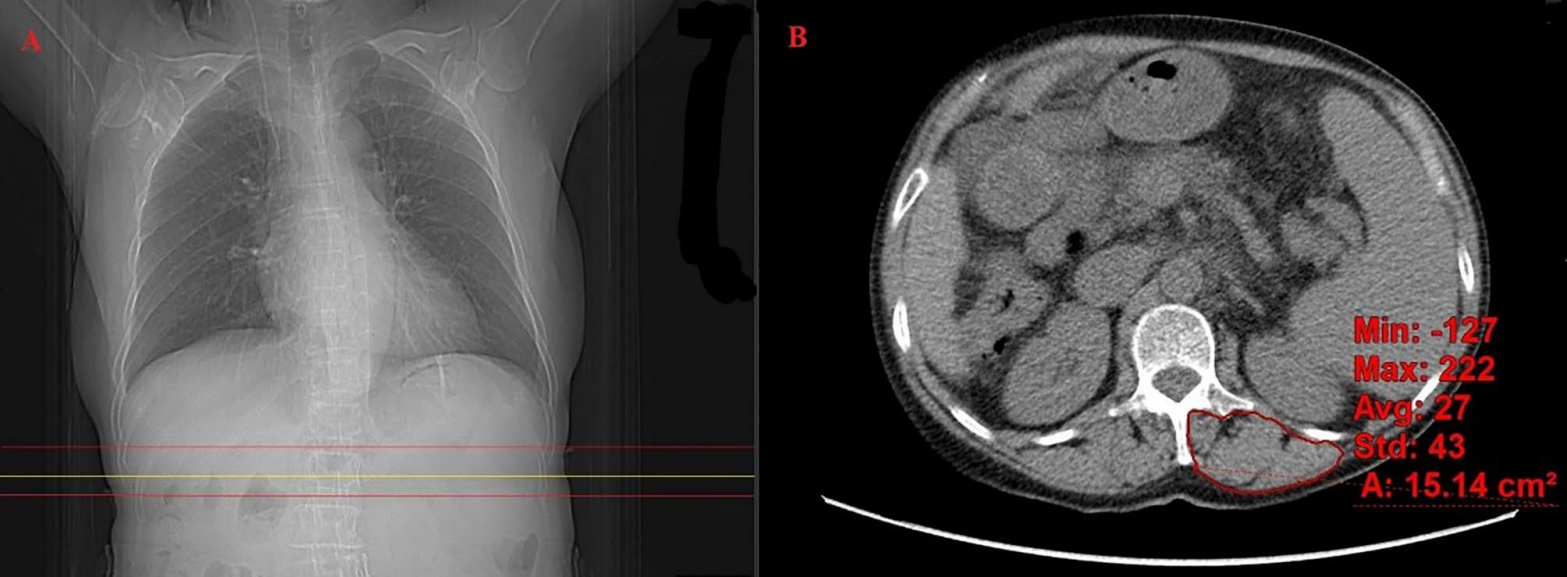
(A) CT in the coronal plane of a patient displaying the levels of muscle examination of different methods (yellow line: CT positioning line; upper red line: upper boundary at L1; lower red line: lower boundary at L1); (B) Exemplary CT of the cross-section of the erector spinal muscles in a patient. The image detail outlined by the red line depicts the detected erector spinal cross-sectional area. L1: the 1st lumbar vertebra
